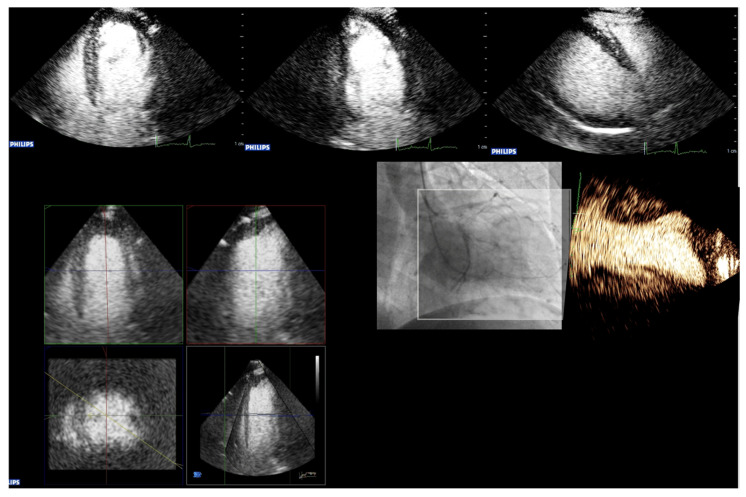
Three-dimensional echocardiography may be useful to intercept abnormalities in non-standard scan planes, as this anterolateral aneurysm would be fully missed if using only standard 4-, 2-, and 3-chamber views. The top row shows the standard apical views obtained by 2D contrast echocardiography. The quad screen on the left shows the standard reconstructed 2D planes from 3D contrast echocardiography. The anterolateral aneurysm is only displayed on a non-standard view (colorized frame mid-right).
